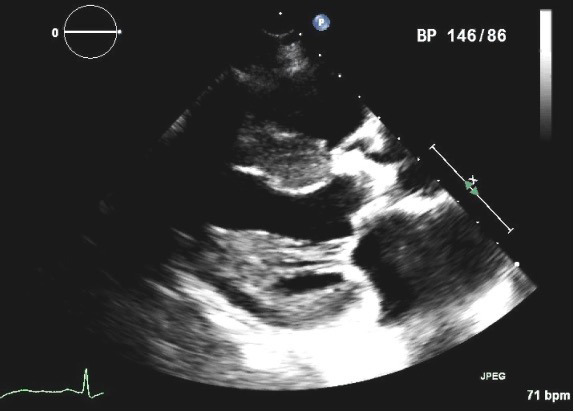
Parasternal long axis view of a four year old 21 mm St Jude
